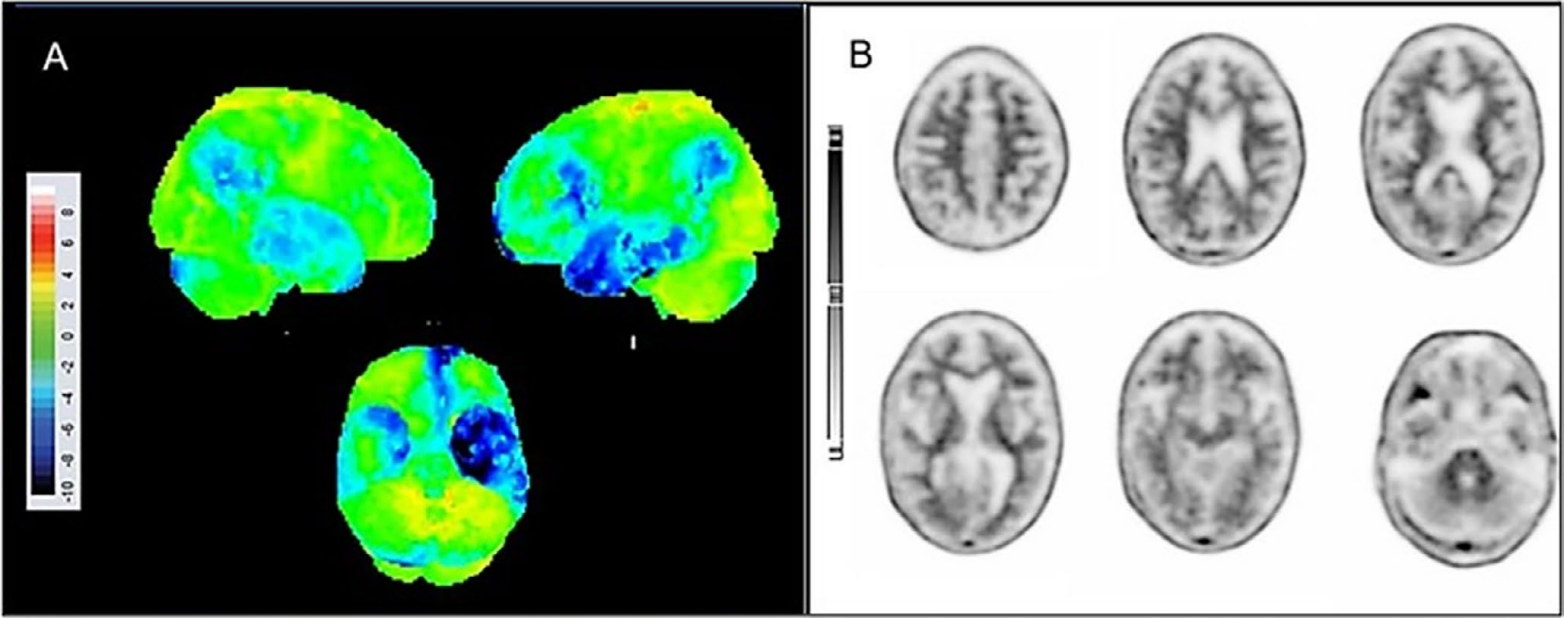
[^18^F]‐FDG PET and amyloid PET images. (A) Statistical surface projections of [^18^F]‐FDG‐PET after database comparison with a normal population adjusted by age. Significant cortical hypometabolism (> 2 SD in blue) is observed in both temporal poles, with a predominance in the left temporal pole, as well as in the left frontal and fronto‐insular regions. (B) [^18^F]‐Florbetapir amyloid PET scan was negative for elevated β‐amyloid cortical deposition (−30.7 Centiloids).
